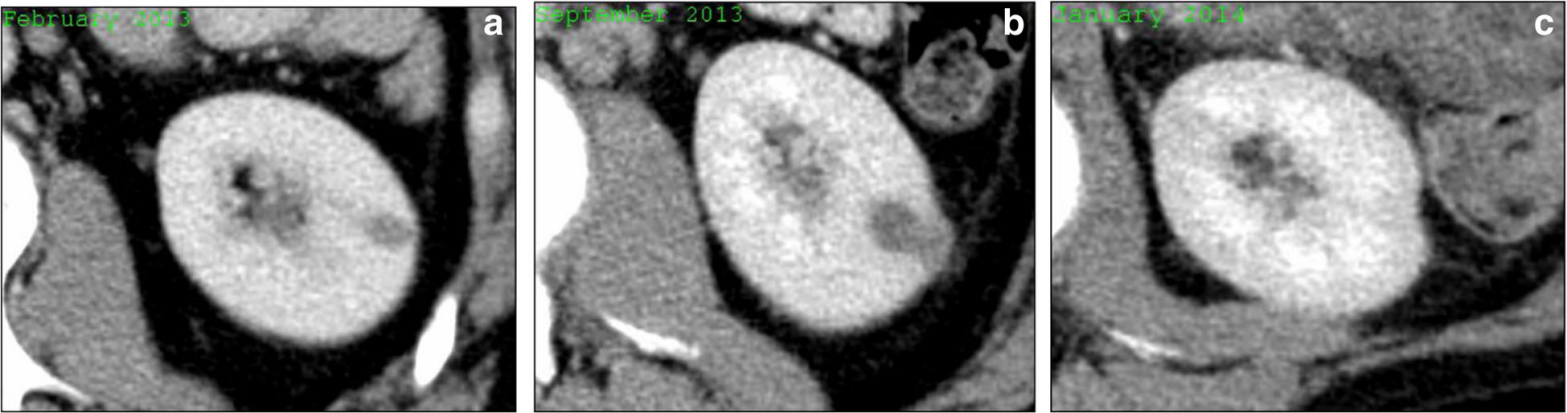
Pattern of evolution of one of the CARCs in patient 1: Axial CT images of the left kidney show enlargement of a 9 mm cyst in patient 1 that was present at baseline in February 2013 (a) to 17 mm in September 2013 (b) followed by spontaneous resolution leaving a cortical scar in January 2014 (c) with ongoing crizotinib therapy
